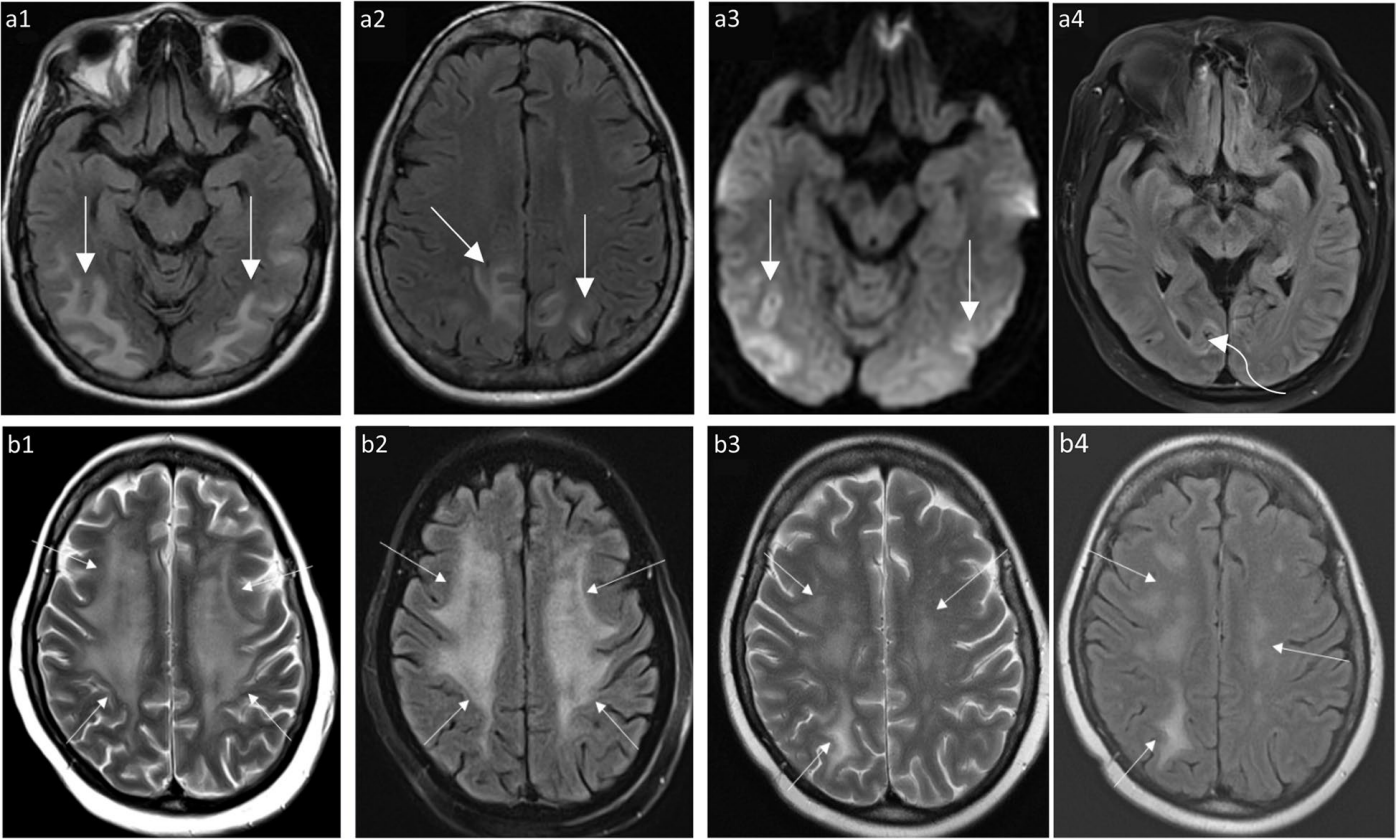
Examples of posterior reversible encephalopathic syndrome (PRES) related to chemotherapeutic agents. a A 52-year-old female with stage IV gallbladder carcinoma underwent a cisplatin-based chemotherapy regimen. Following the third cycle, she experienced worsening headache and giddiness, leading to hospital admission after two episodes of generalized tonic–clonic seizures. Axial FLAIR images (a1, a2) display hyperintensities in bilateral parietal, occipital, and posterior temporal lobes. The corresponding axial DWI image (a3) reveals increased signal and low apparent diffusion coefficient (ADC) (not shown), suggestive of PRES. A follow-up axial FLAIR image (a4) six weeks post-chemotherapy cessation shows near-complete resolution, with only residual signal in the right lingual gyrus (curved arrow). b A 45-year-old female with triple-negative breast cancer (TNBC) underwent mastectomy and received a Bevacizumab-based chemotherapy regimen. Axial T2 (b1) and FLAIR (b2) MRI brain images exhibit symmetrical hyperintensities in bilateral centrum semiovale and corona radiata (arrows), indicating Bevacizumab-related PRES. Subsequent MRI post-bevacizumab cessation demonstrates significant improvement in signal abnormalities on axial T2 (arrows, b3) and FLAIR (arrows, b4) images
